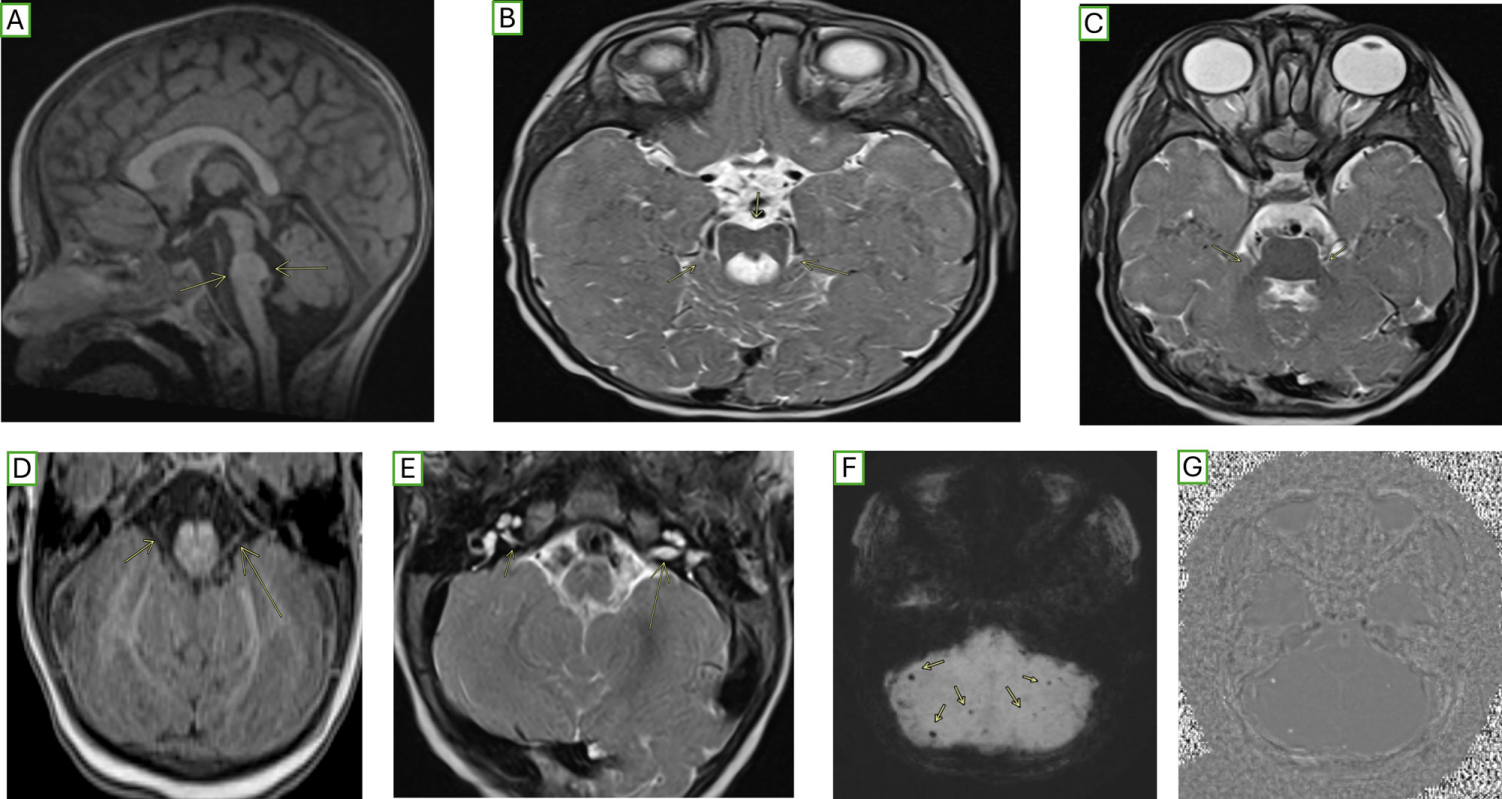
Midline sagittal T1-weighted imaging (A) demonstrates significant ventral flattening of the pons, with dorsal rounded fullness protruding into the fourth ventricle. Axial T2-weighted imaging at the level of the isthmus (B) reveals the classic “molar tooth” configuration, characterized by laterally displaced superior cerebellar peduncles and a deep interpeduncular fossa. Additional axial T2-weighted imaging (C) shows hypoplasia of the middle cerebellar peduncles. Axial T1-weighted imaging without contrast (D) depicts a smaller right cranial nerve VII/VIII complex; however, no high-resolution T2 images were available for further evaluation. Another axial T2-weighted image (E) highlights a smaller right internal auditory canal compared to the left. Finally, axial susceptibility-weighted (F) and phase (G) imaging demonstrate several foci of susceptibility artifact in both cerebellar hemispheres, consistent with prior microhemorrhages, without evidence of mass effect or edema.
